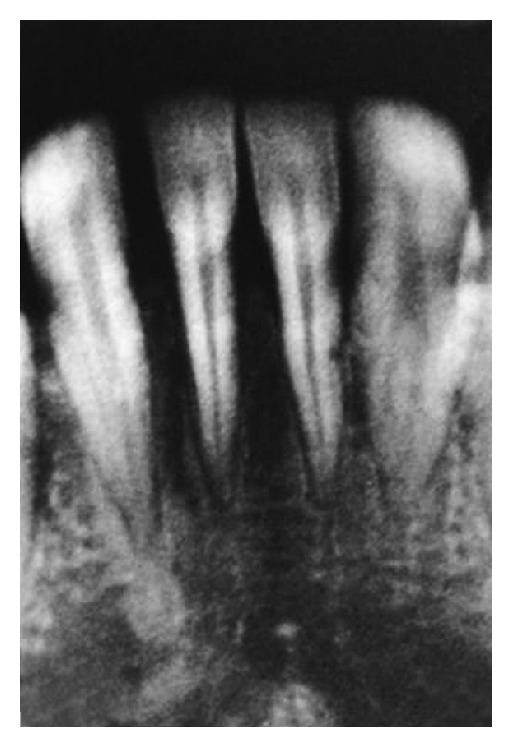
Preoperative radiograph of 41 region with interdental bone loss.
